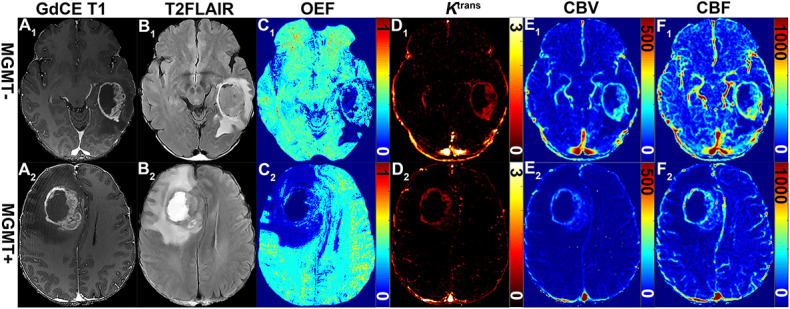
Representative MRI-based OEF and DCE perfusion images of glioma patients with and without methylated MGMT promoter. Two GBM patients with unmethylated (51-year-old woman with MGMT^–^: A_1_–F_1_) and methylated (58-year-old man MGMT^+^: A_2_–F_2_) promoter shown: (A_1_) GDCE 3D T1BRAVO, (B_1_) T2-FLAIR, (C_1_) QQ-based OEF maps with CAT, DCE-MRI with separated *K*^*trans*^
